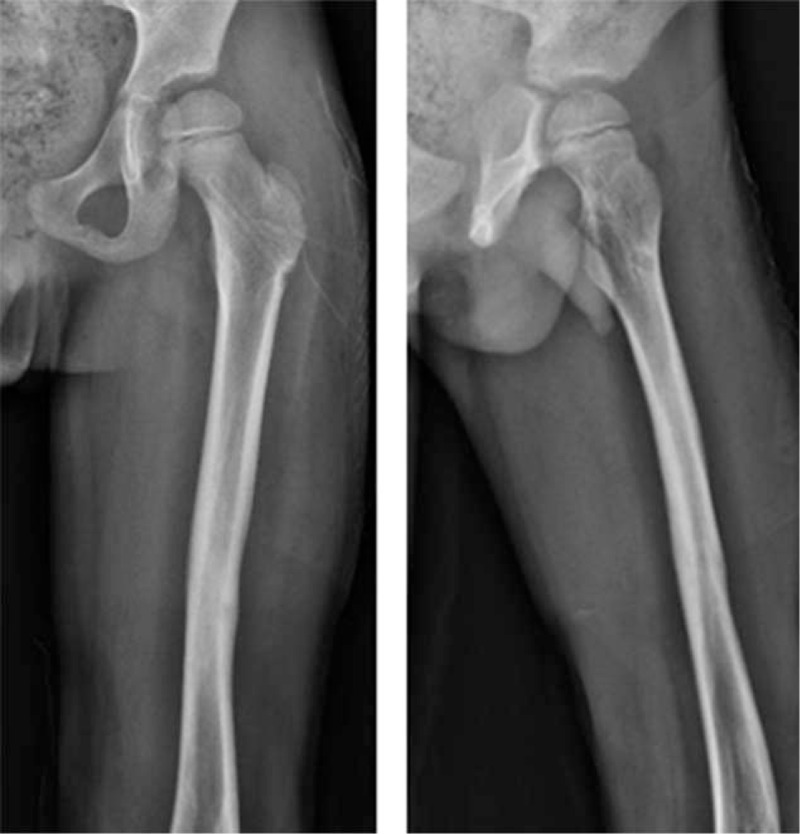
The above pictures are the left femur X-ray films after removal of internal fixation 1 year after operation.
